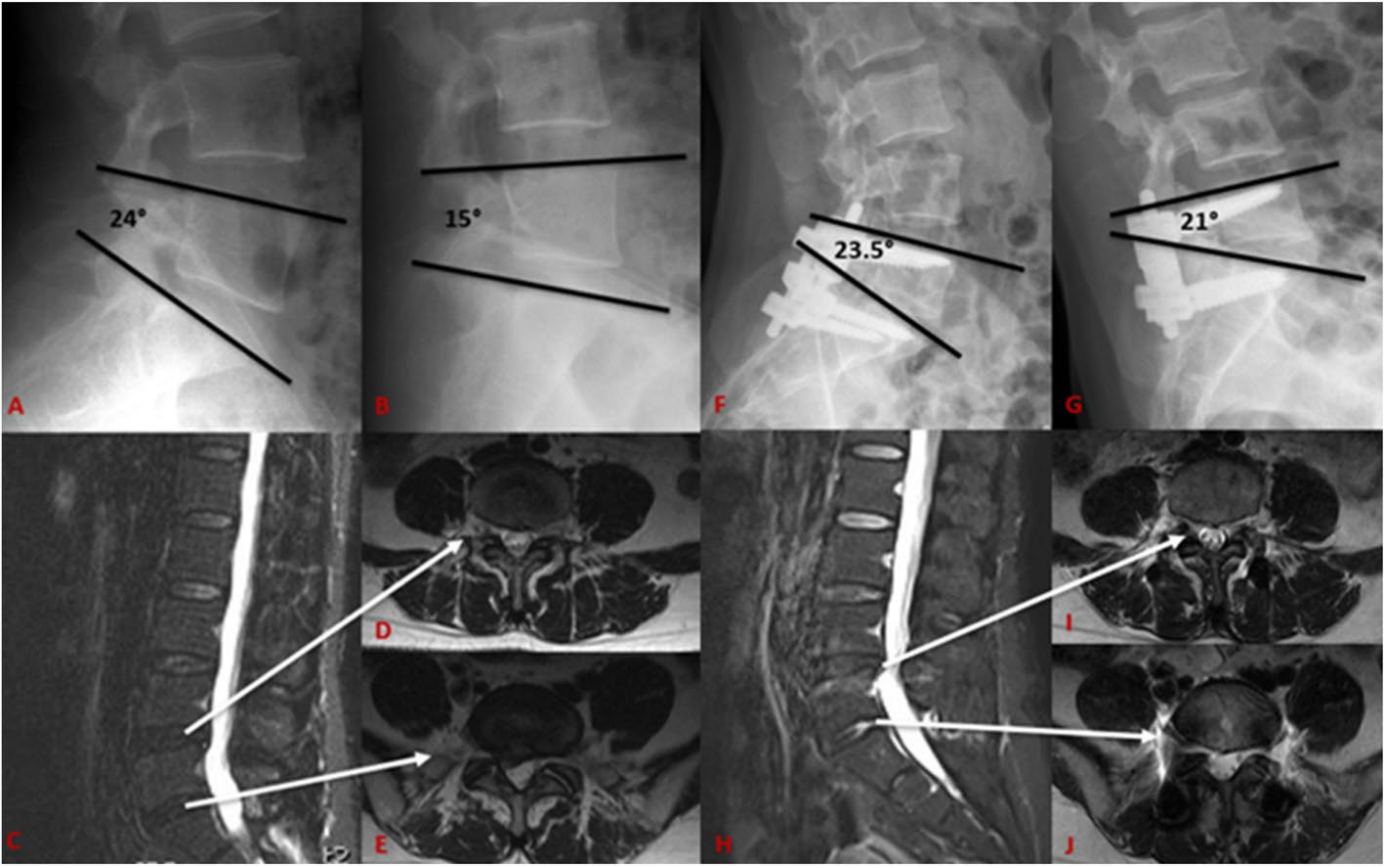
A 48-year-old woman had disc herniation at L5–S1, and the ROM of the surgical segment was 9° based on X-ray (A-B). The MRI of pre-operation showed that the disc signal of L4/5 and L5/S1 (C-E). She underwent fenestration decompression and posterior TTL dynamic fixation at L5-S1. The radiographs obtained 49 months after the operation showed the operative segment’s ROM was 2.5°, and the L4/5 ISH was slightly lost compared to preoperative. The L5/S1 ISH did not change significantly compared to preoperative, the L4/L5 disc signal was good, but there was slight bulging, the degree of spinal stenosis did not increase significantly compared to the pre-operation, and the UCLA classification changed from grade I to grade II. And there was no significant difference in the L5/S1 intervertebral disc signal from before surgery (F-J)
